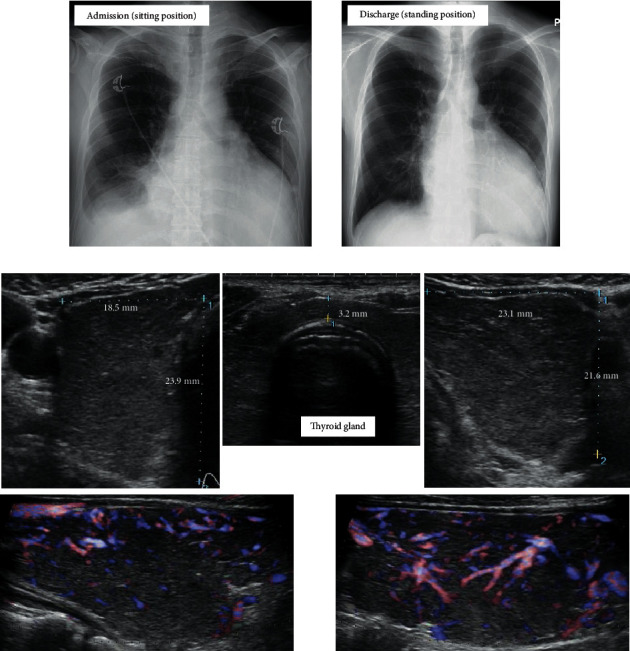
Imaging findings at first admission. (a) Chest X-ray on admission and discharge. (b) An ultrasonogram showing enlargement and increased blood flow of the thyroid gland.
